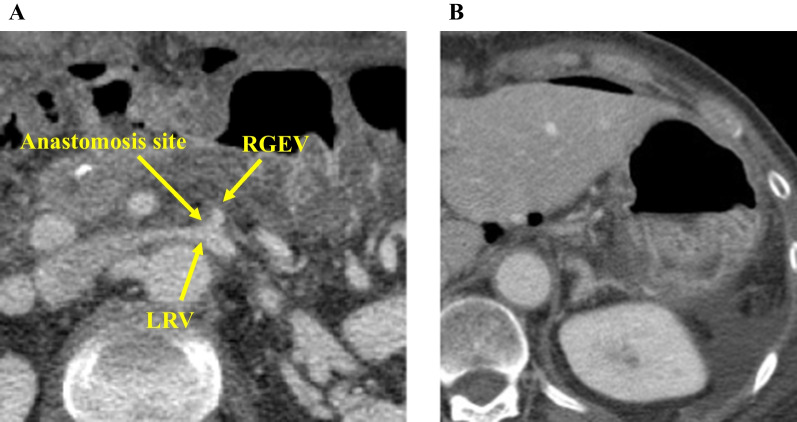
CT after the surgery. A CT 6 days after the surgery showing the patency of the RGEV–LRV anastomosis. B CT 6 days after the surgery showing the absence of varices around the stomach. *CT* Computed tomography, *RGEV* Right gastroepiploic vein, *LRV* Left renal vein
